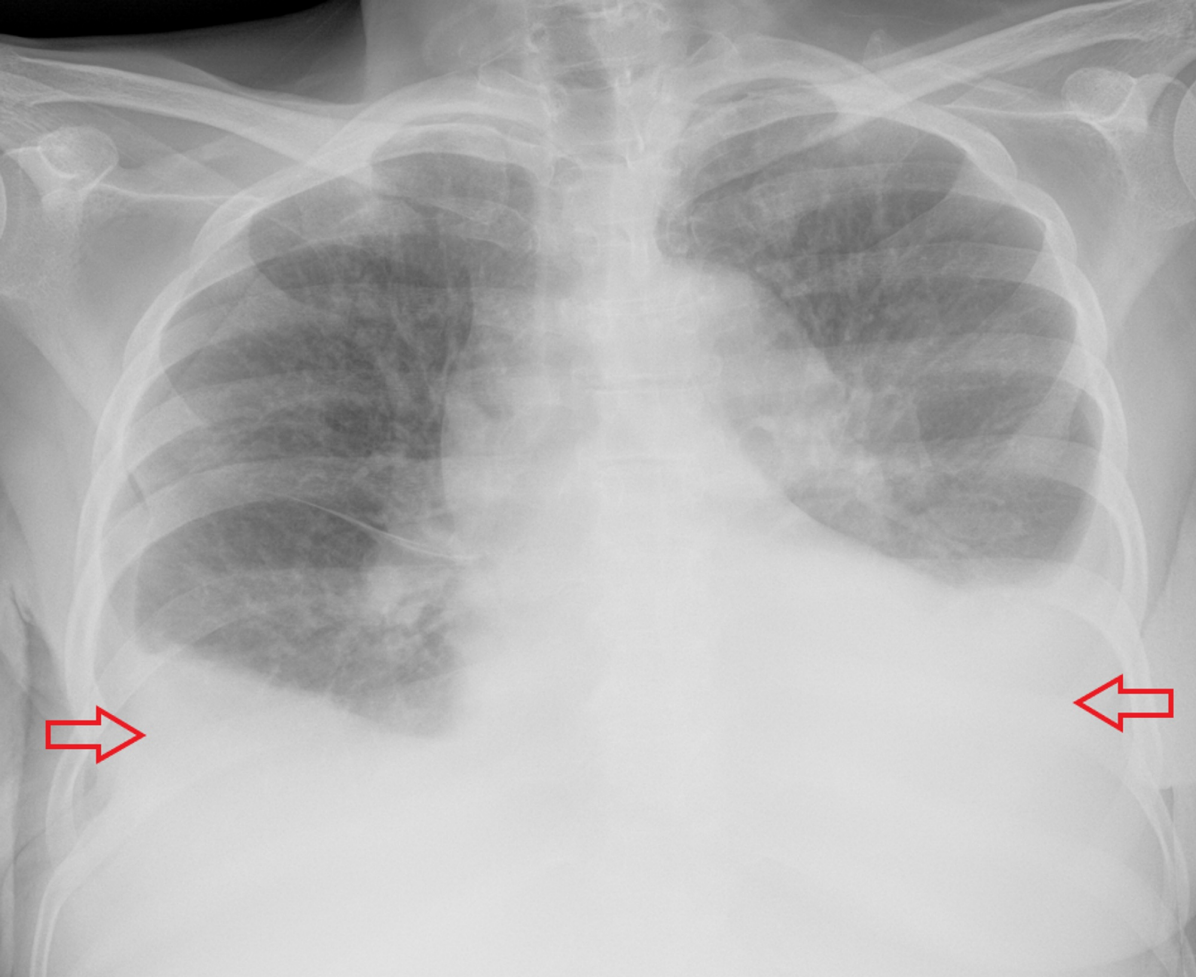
Chest X-ray showing bilateral pleural effusion with bibasilar atelectasis
